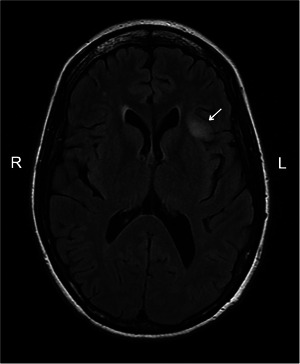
Low‐grade glioma imaged with an axial fluid‐attenuated inversion recovery (FLAIR) MRI scan. White arrow points at the low‐grade glioma (high FLAIR signal) in the left anterior insula. L, left; R, right.
